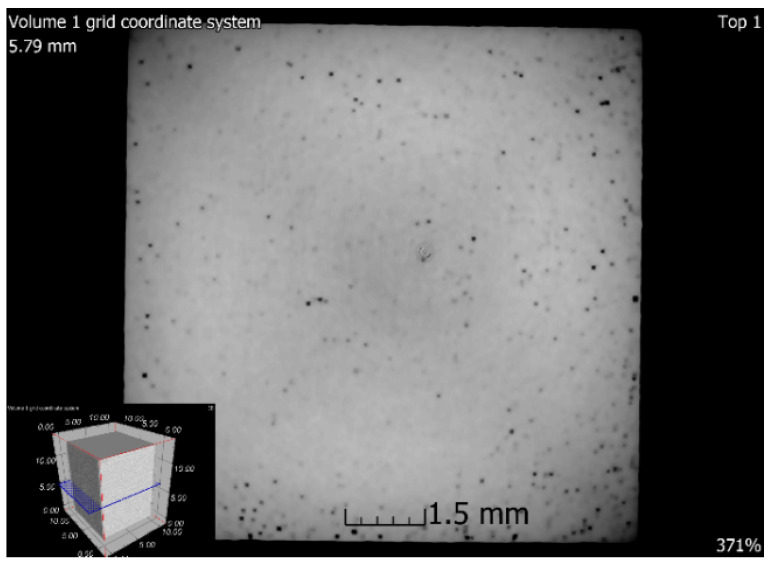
Tomographic CT sections of sample 5 with an adaptive Gaussian filter.
